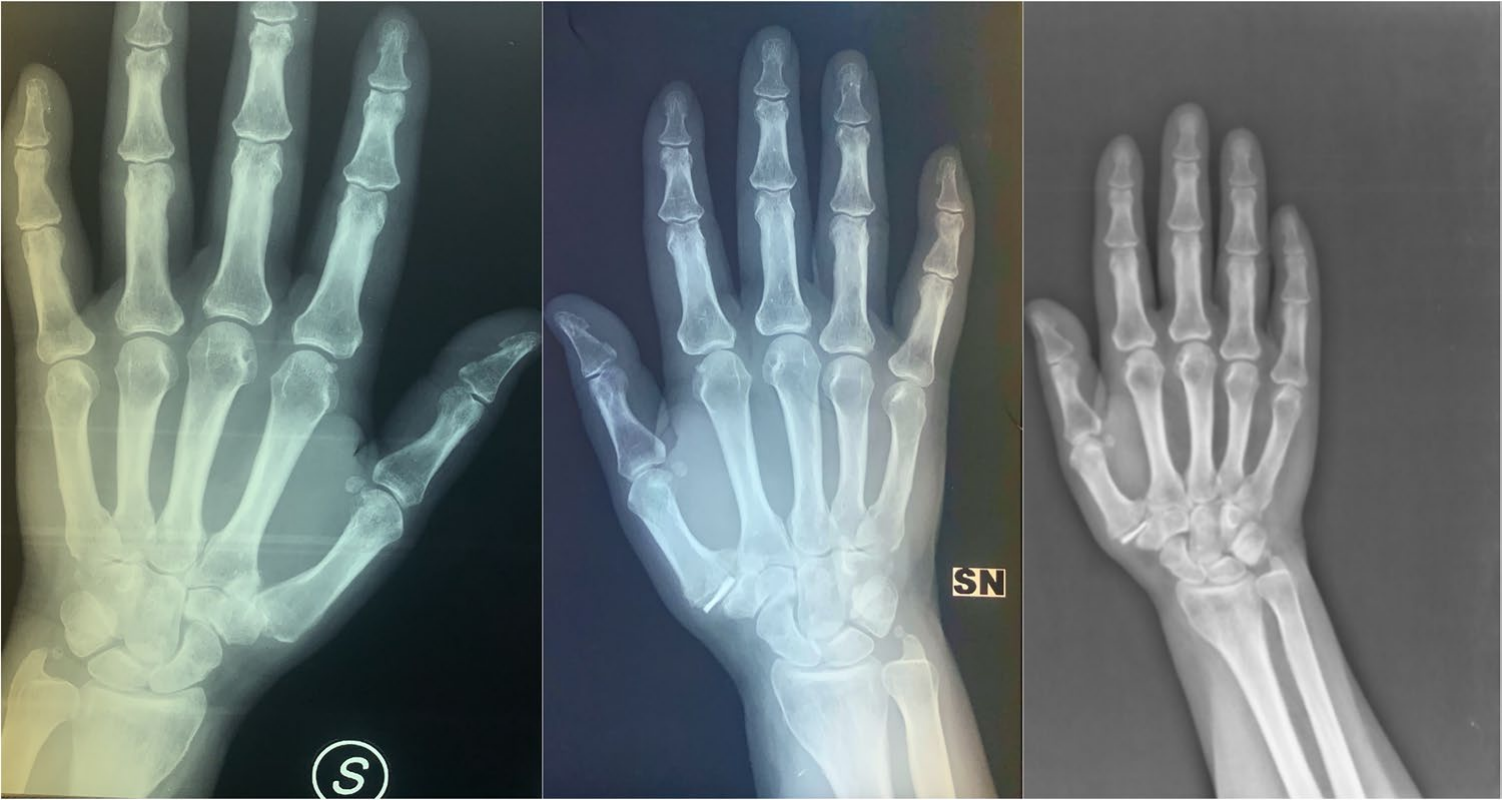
Woman, 64 years old, pre-operative X-ray, post-operative X ray at 3 months and post-operative X-ray at last follow-up of 5 years
